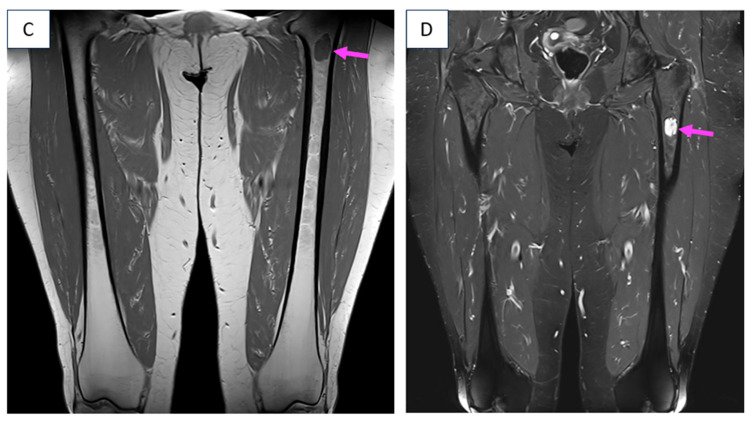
Later on, at the age of 57, the patient experienced non-specific, mild, persistent bone and joint pain and further investigations lead to the identification, not only of incipient arthrosis, but, also, of a left femoral tumor with cartilaginous structure and benign appearance (an enchondroma) at computed tomography (CT) and magnetic resonance imaging (MRI) [(A,B) CT scan (native phase): bone tumor in the diaphysis of the left femur of 1.9 by 2.5 cm, without cortical involvement (pink arrow); (A) coronal plane; (B) coronal plane with bone window. (C,D) Contrast-enhanced MRI scan: bone tumor in the diaphysis of the left femur of 1.9 by 1.8 by 2.9 cm with polycyclic margins, with T1 hypo-signal and T2 hypersignal, without cortical thinning, suggestive of enchondroma (pink arrow); (C) T1 WI HSE, coronal plane; (D) T2 FSE, coronal plane].
